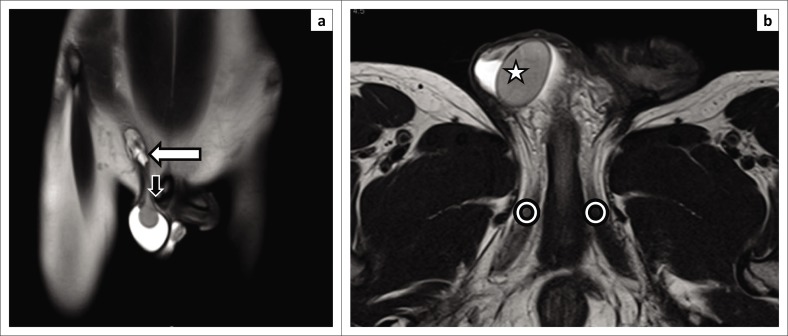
(a) Coronal and (b) axial T2-weighted (T2W) magnetic resonance images in 9-month-old male child showing true undescended right testis (star) at the level of the root of penis, near the pubic tubercle (black arrow). The crura of the penis are seen at this level (circles). The inguinal canal (white arrow) is well visualised on coronal section.
